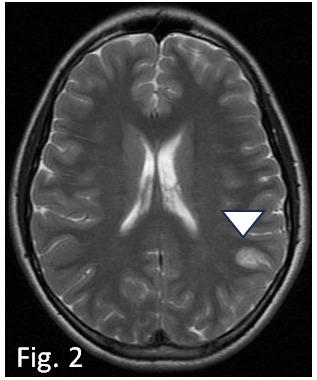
Axial FSE T2W MRI performed one day after the debut, shows
